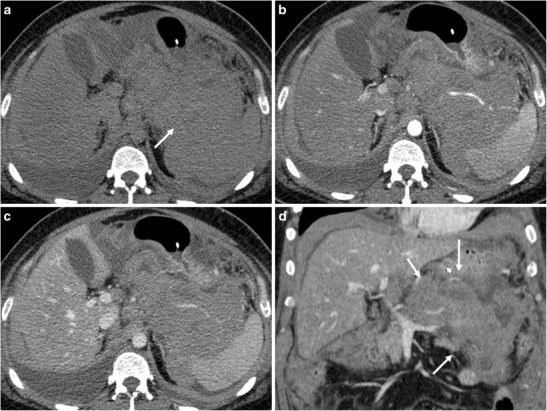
A 42-year-old male patient with primary pancreatic lymphoma, histotype diffuse large B-cell lymphoma (Ki 67 score > 95%). a. CT scan of the abdomen shows the presence of a large solid mass involving the pancreatic body and tail (arrow); the lesion reaches the spleen and almost encases it. b, c CT images after contrast medium administration in the arterial-pancreatic phase (a) and portal-venous phase (b): the neoplasm is hypodense, with progressive contrast enhancement. d. This coronal CT reconstruction allows better appreciation of the size and extension of the lesion (arrows)
